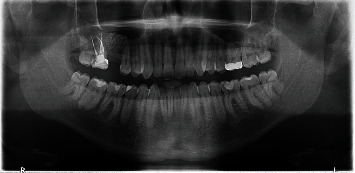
Panoramic radiograph. Preoperative situation before implant placement.
